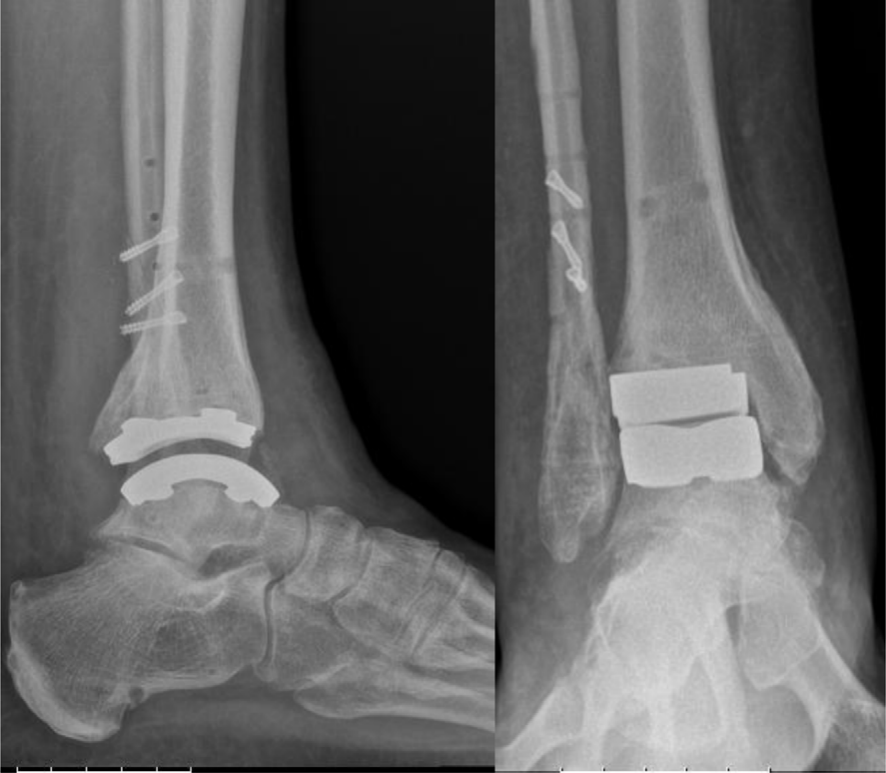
Lateral and antero-posterior view of the weight-bearing control radiographs.
